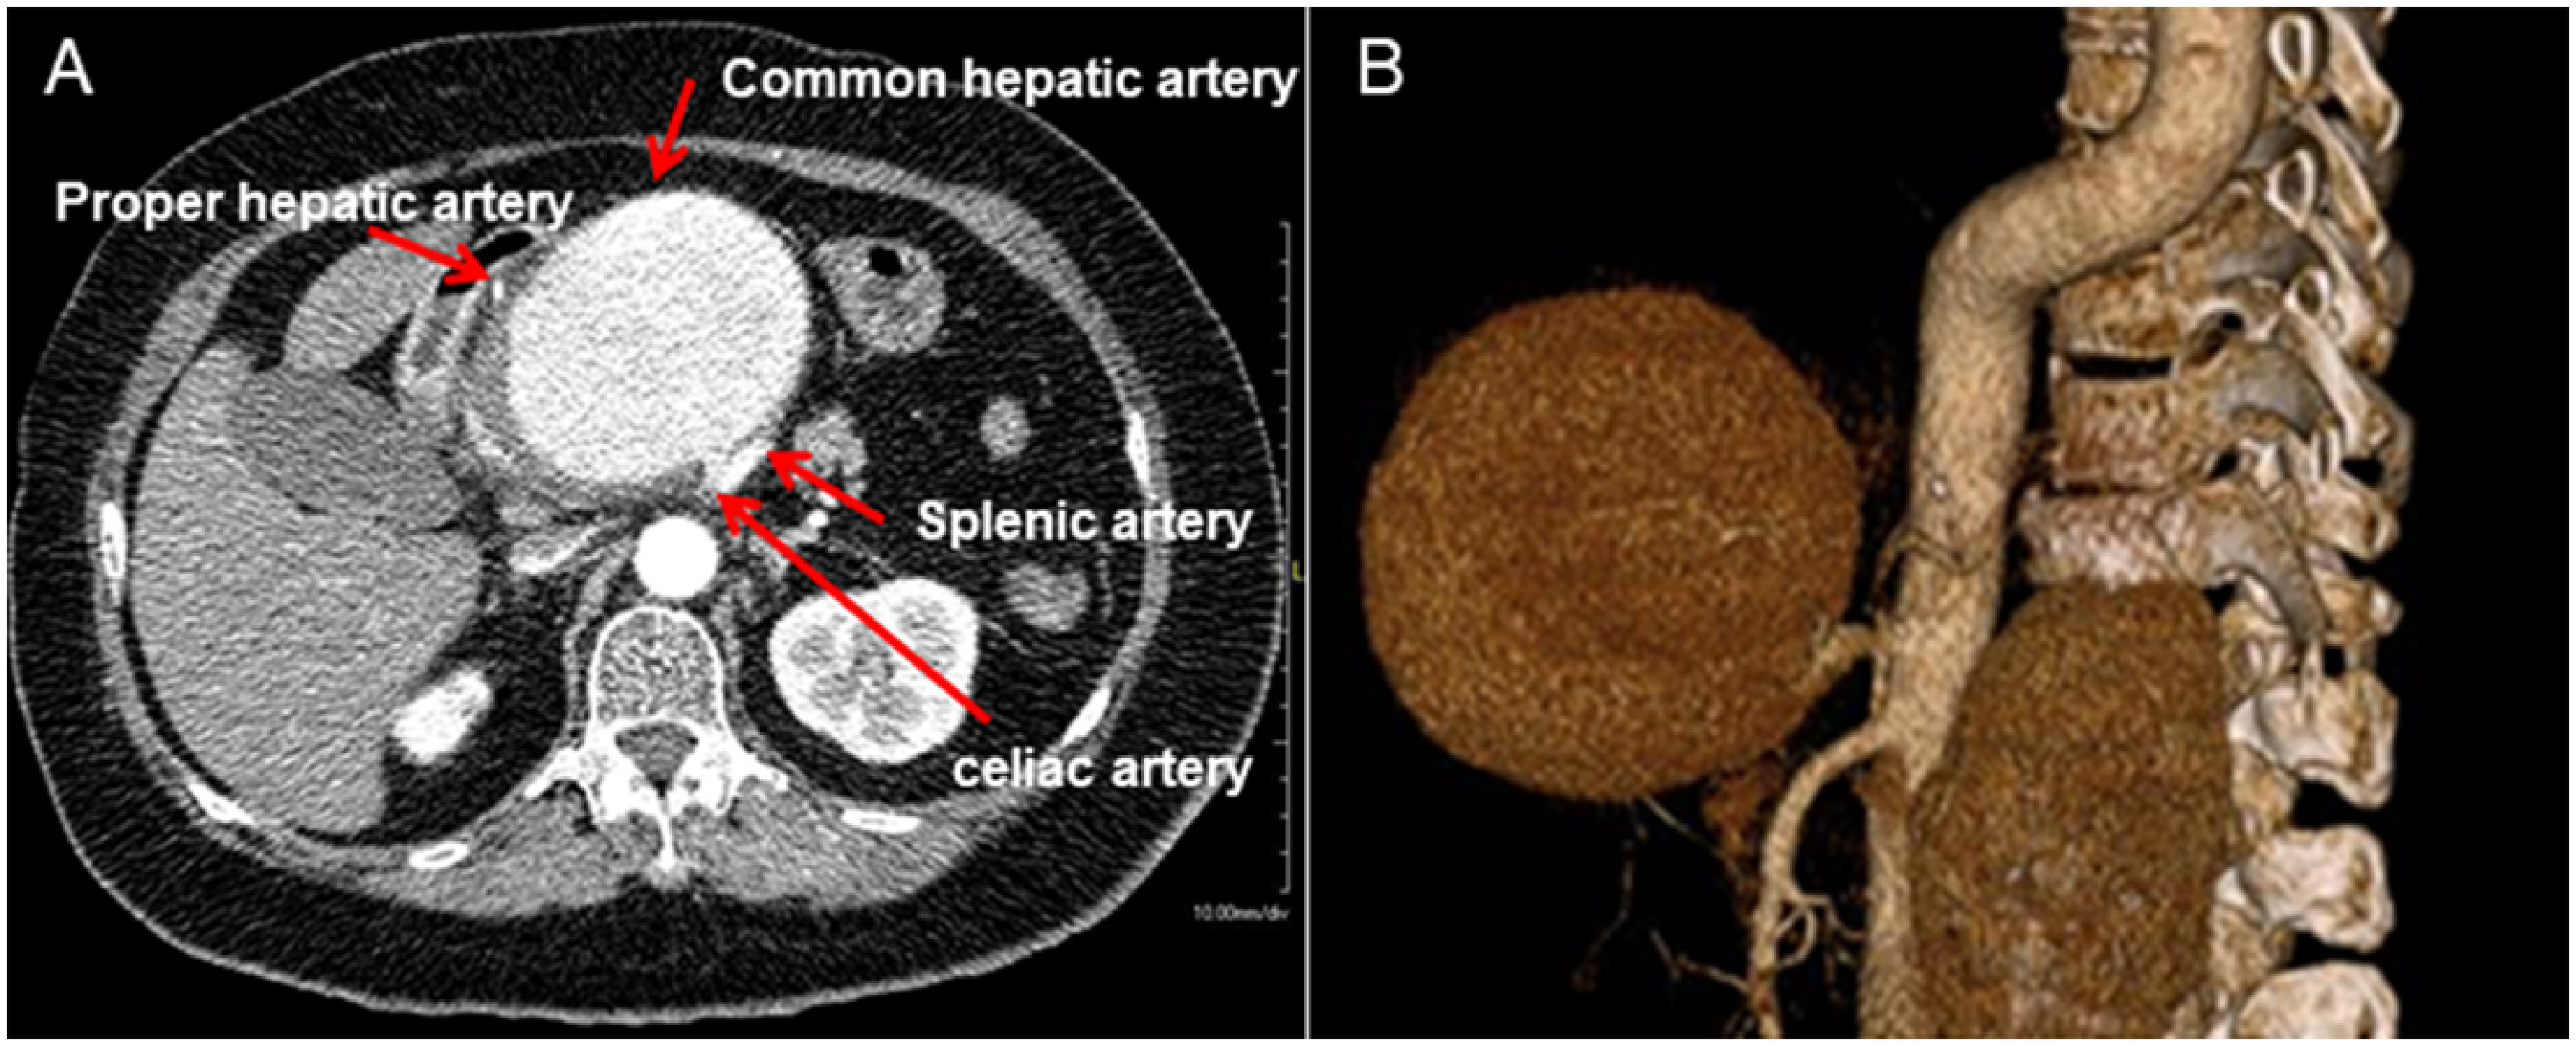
Fig. 1 (A) Computed tomography image showing the hepatic artery aneurysm. The size of the aneurysm was 11×9 cm. The orifice of inflow into the aneurysm started 12 mm distal to the origin of the celiac artery after branching of the splenic artery. The proper hepatic artery and gastroduodenal artery ran very close to the aneurysm. (B) Three-dimensional computed tomography image of the aneurysm.
